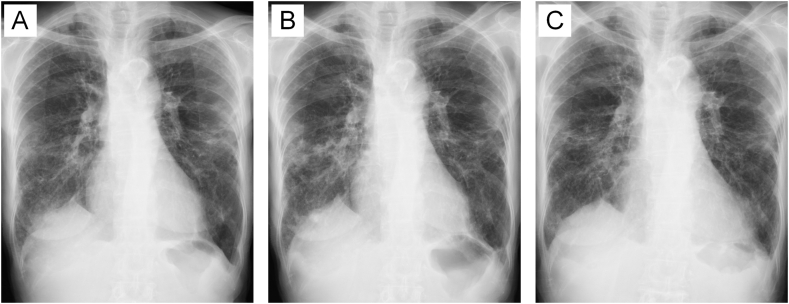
Clinical course of Case 1. Compared to six months prior [A], chest X-ray at the initial detection of *Scedosporium* showed increased infiltration in the right lung [B]. Two years and eight months later following re-treatment for MAC-PD, the chest X-ray demonstrated improvement despite persistent positive cultures for *Scedosporium* [C].
